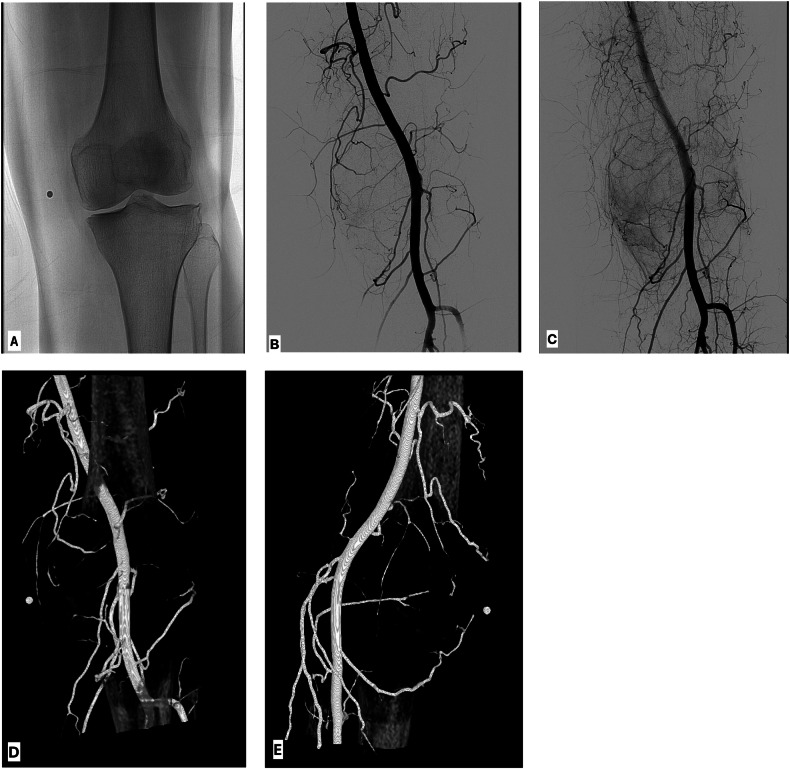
Intra-procedural imaging during GAE. A Scout radiograph demonstrated moderate medial joint space narrowing and mild endplate changes (KL grade 3). A radiopaque marker identifies the area of pain along the medial joint space. B DSA from the superficial femoral artery. C Delayed images from DSA demonstrate hypervascularity along the medial joint space corresponding to the area of pain. D Three-dimensional AP and (E) lateral reconstructions from intra-procedural cone beam CT demonstrate branches of the DGA and inferior medial genicular artery coursing towards the area of pain along the medial joint space, as indicated by radiopaque marker
